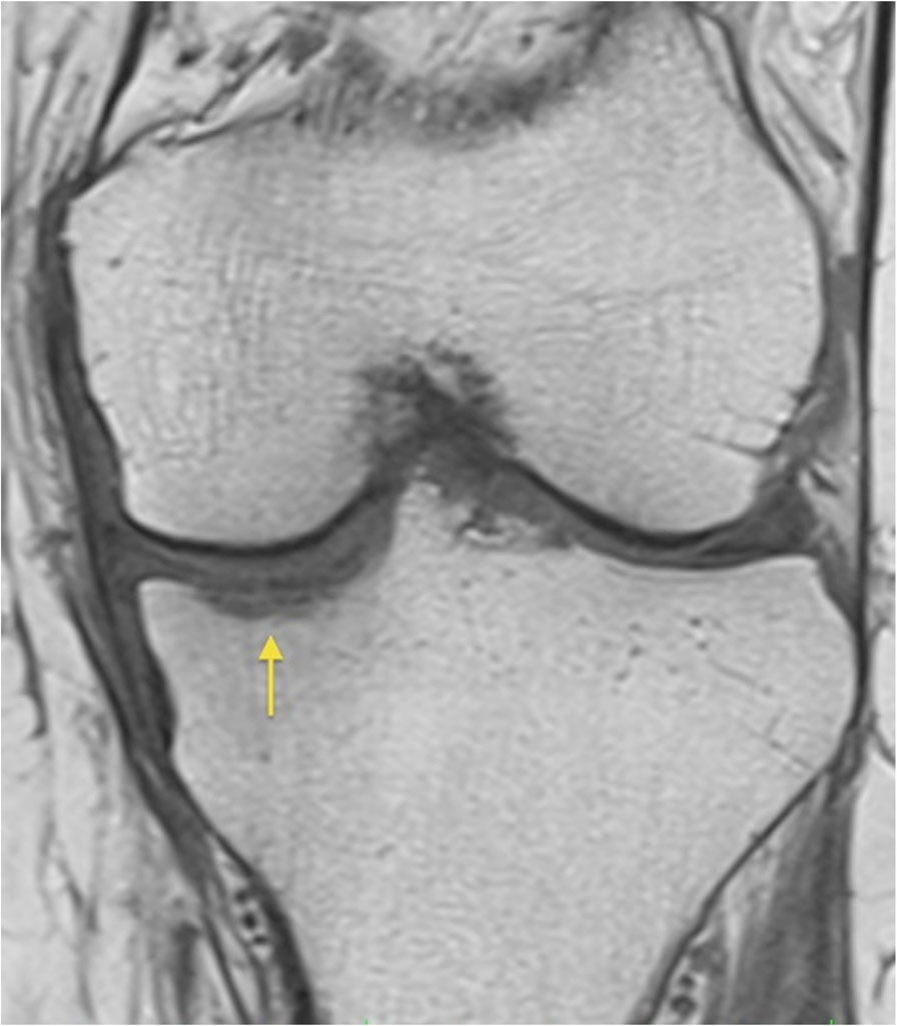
Case 1 patient: preoperative T1-weighted magnetic resonance imaging left knee: underneath the articular surface linear subchondral insufficiency fracture (*arrow*)
